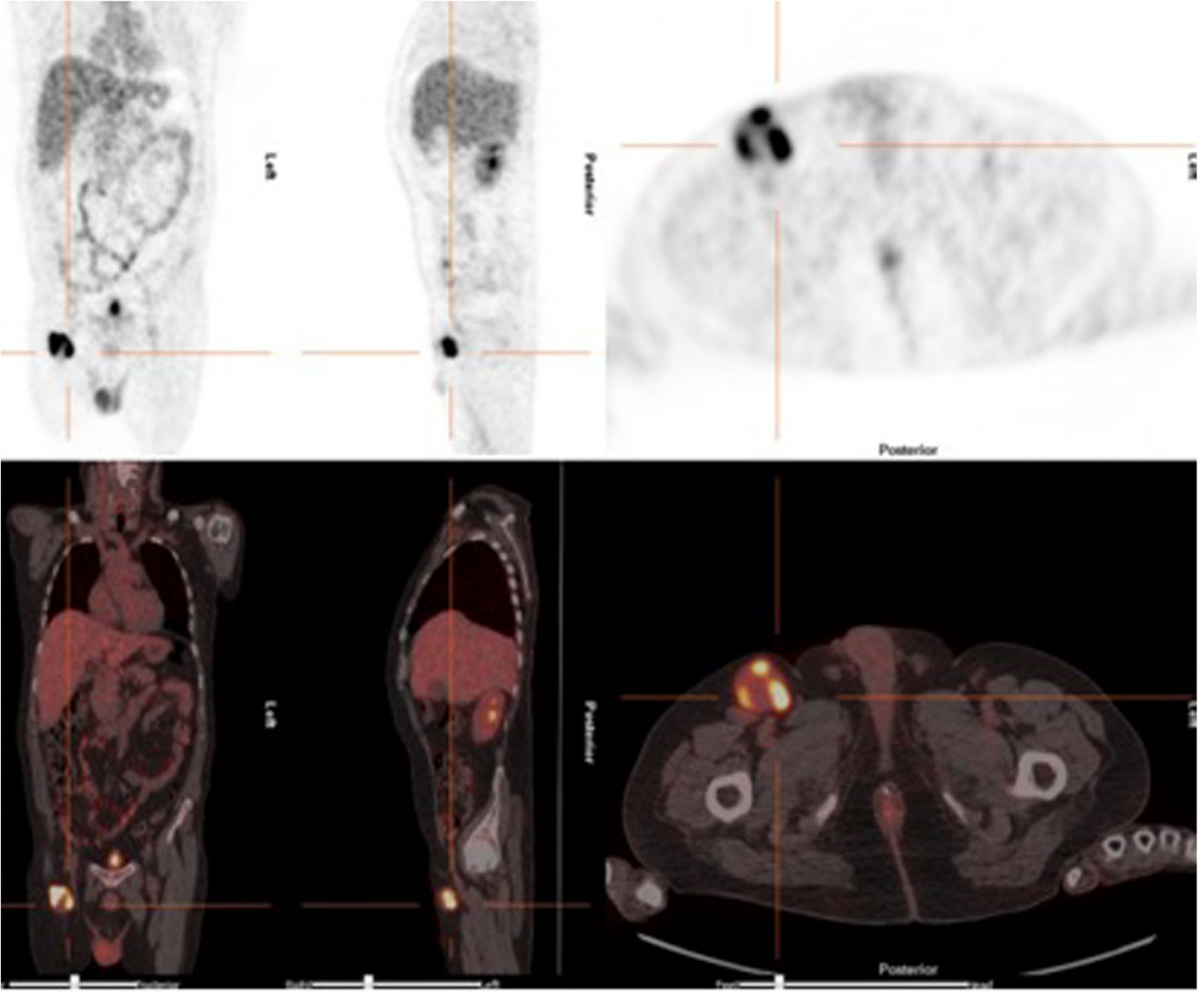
The 18F-FDG PET/CT revealed a pathological deposit in one inguinal lymph node at the right side. The dimension of the lymph node was 4.2 cm × 3.8 cm × 3.5 cm. No other metastases
